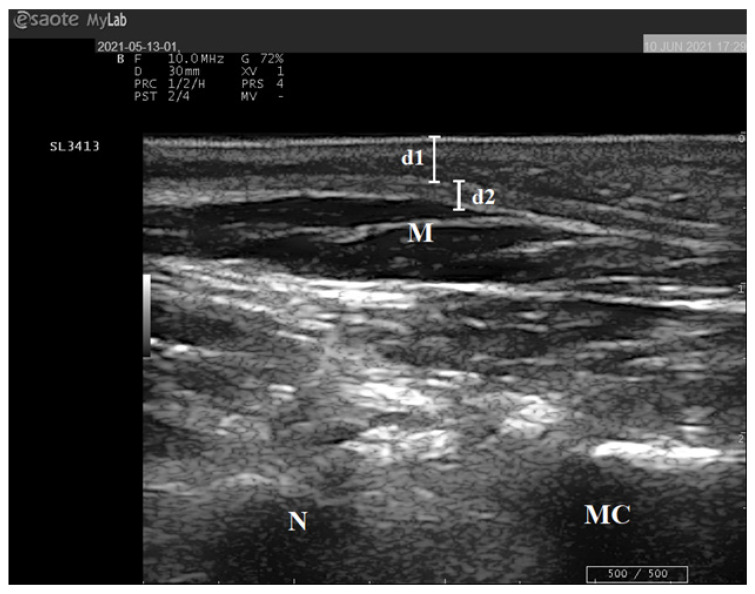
Ultrasound of the medial midfoot plantar surface in an eight-year-old healthy girl. (d1): the thickness of MMFP; (d2): the thickness of MPF; (M): short flexor muscle of the fingers; (N): navicular bone; (MC): medial cuneiform bone.
